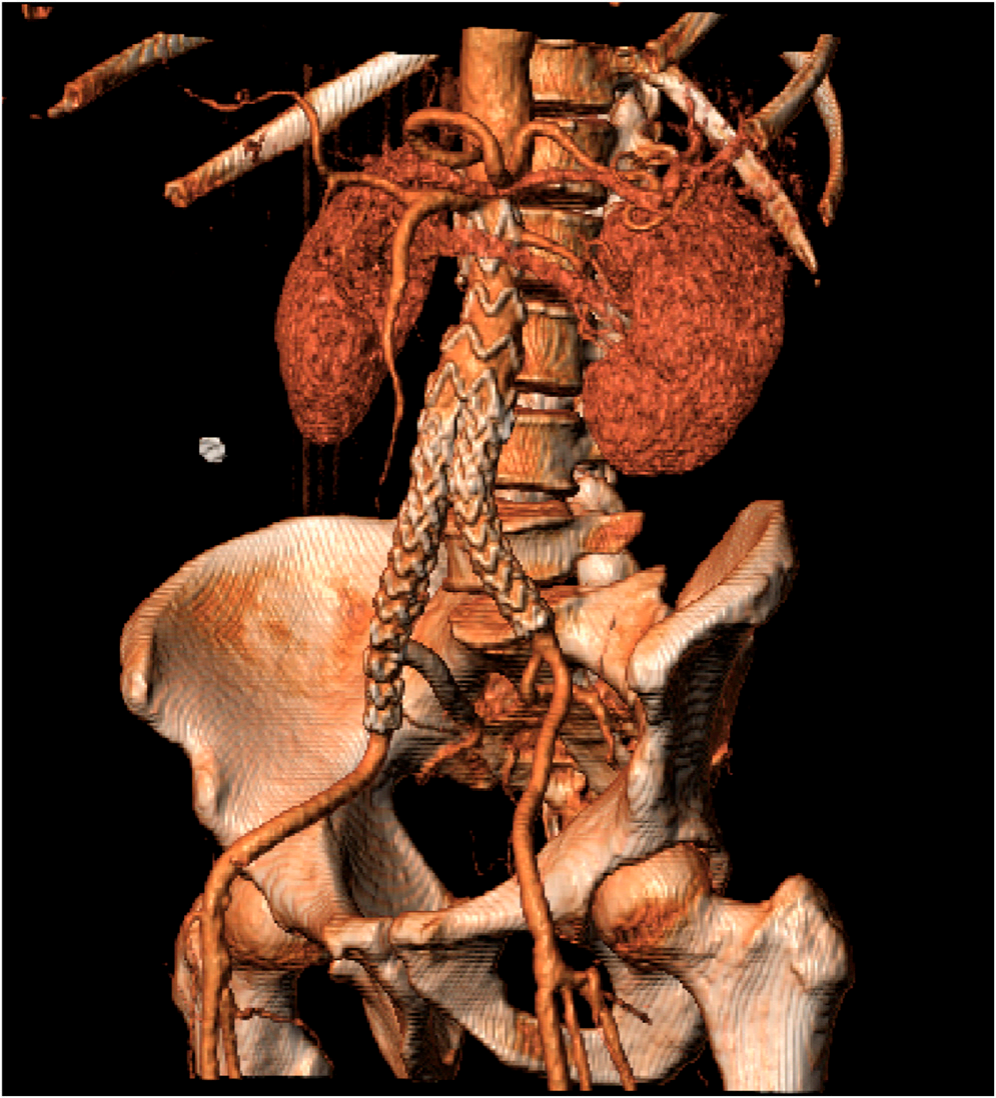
Follow-up computed tomography (CT) scan after 6 weeks (Figure shows reconstruction of the CT-scan. The raw CT-scan showed no signs of endoleak and the right internal iliac artery was patent).
